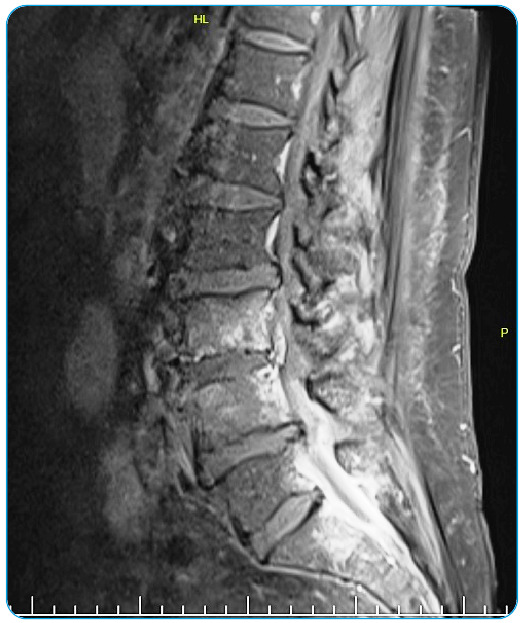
Contrast-enhanced T1-weighted fat saturated magnetic resonance imaging shows L3-4 and S1 spondylodiscitis with epidural enhancement.
